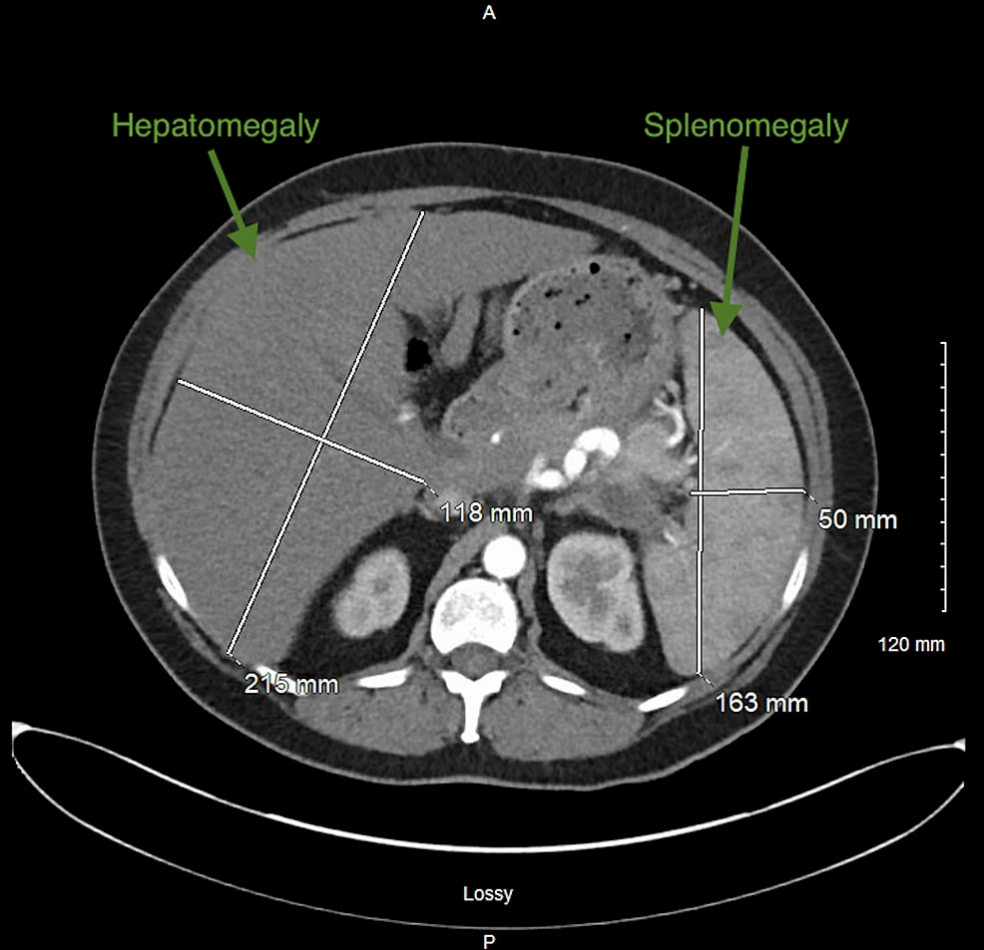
Enhanced computed tomography of the abdomen and pelvis. Liver dimensions: 11.8 x 21.5 x 24.3 cm. Spleen dimensions: 16.3 x 5.0 x 15.9 cm.
